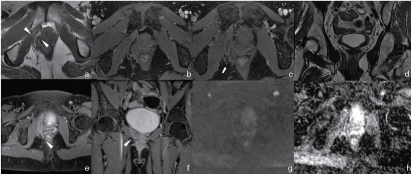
6-month MRI control scan after hormonal replacement therapy withdrawal. (a) Axial *T*
